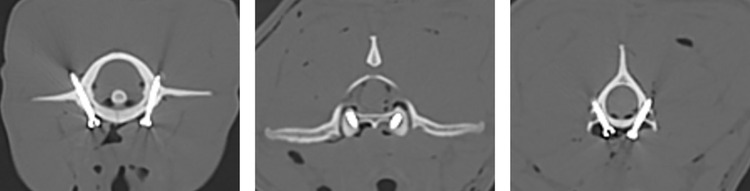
Transverse CT image of C1, C2 cranial, and C2 caudal.
